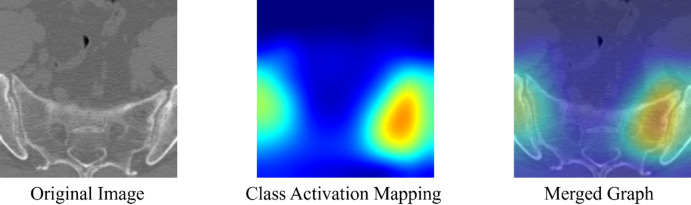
Grad-CAM visualization: The first Image shows the original CT; the second Image shows the Class Activation Map; the third Image shows the Fused Image (Red regions indicate high model attention/activation).
